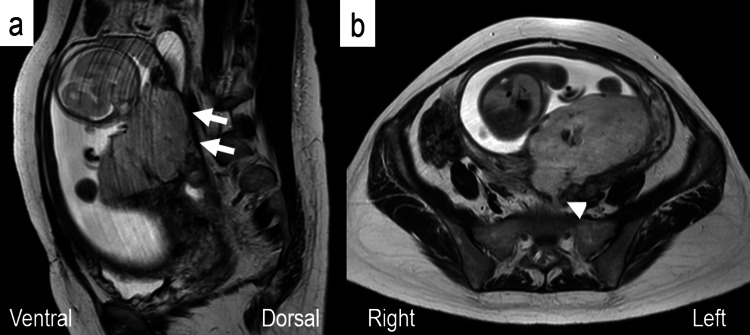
MRI findings at 22 weeks of gestation. (a) T2-weighted MRI revealed that the uterine wall from the bottom to the posterior wall of the uterus was thinning and bulging outward (arrows). (b) A part of the maternal side of the placenta was exposed outside the posterior uterine wall, suggesting the presence of placenta percreta (arrowheads).
